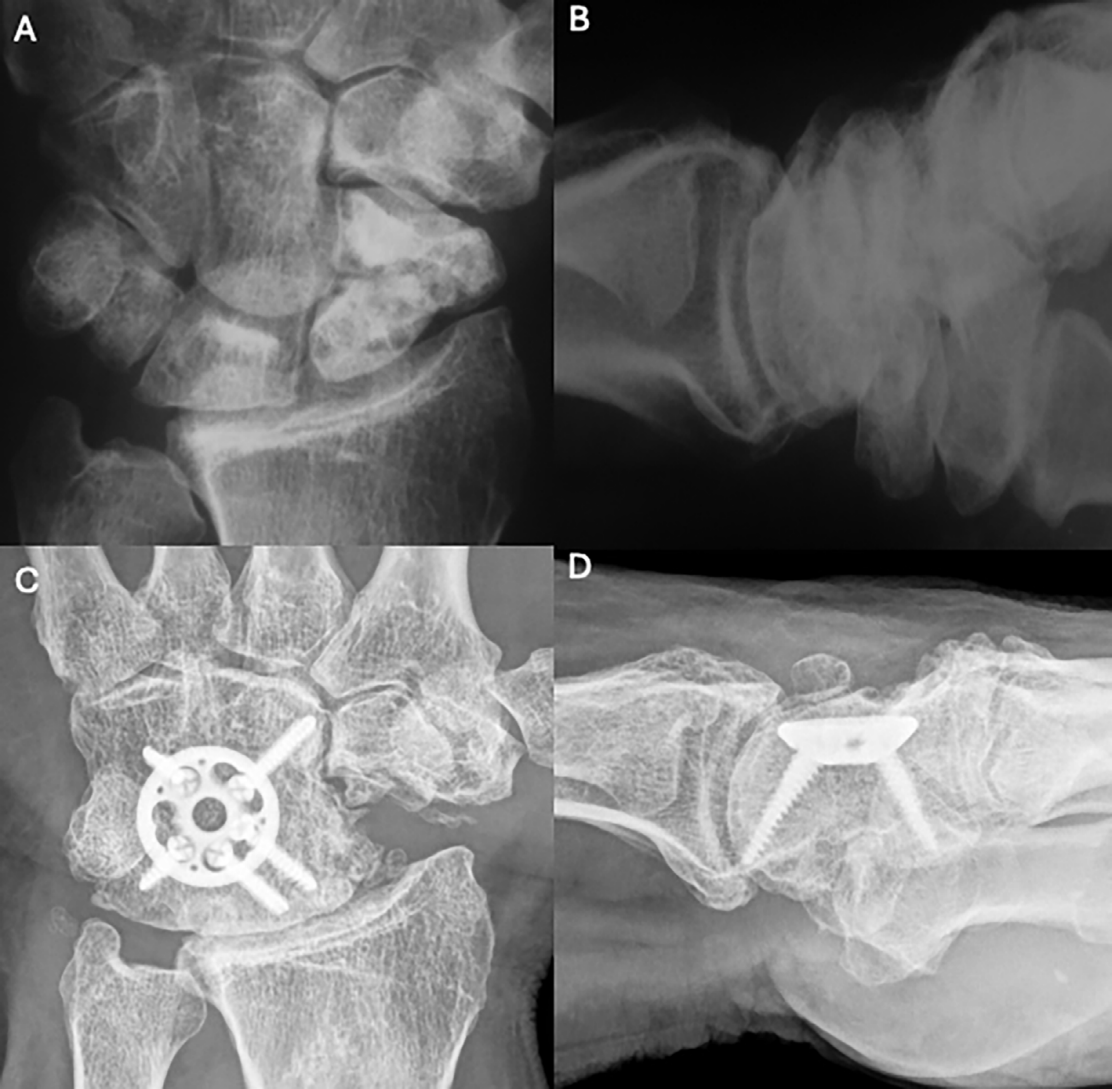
Clinical case of 4-Corner Fusion. A and B. Preoperative wrist X-rays of SNAC stage II (AP and Lateral views). C and D. Postoperative wrist X-rays (AP and Lateral views) after 17 years. Source: Dr. Emmanouil Apergis.
